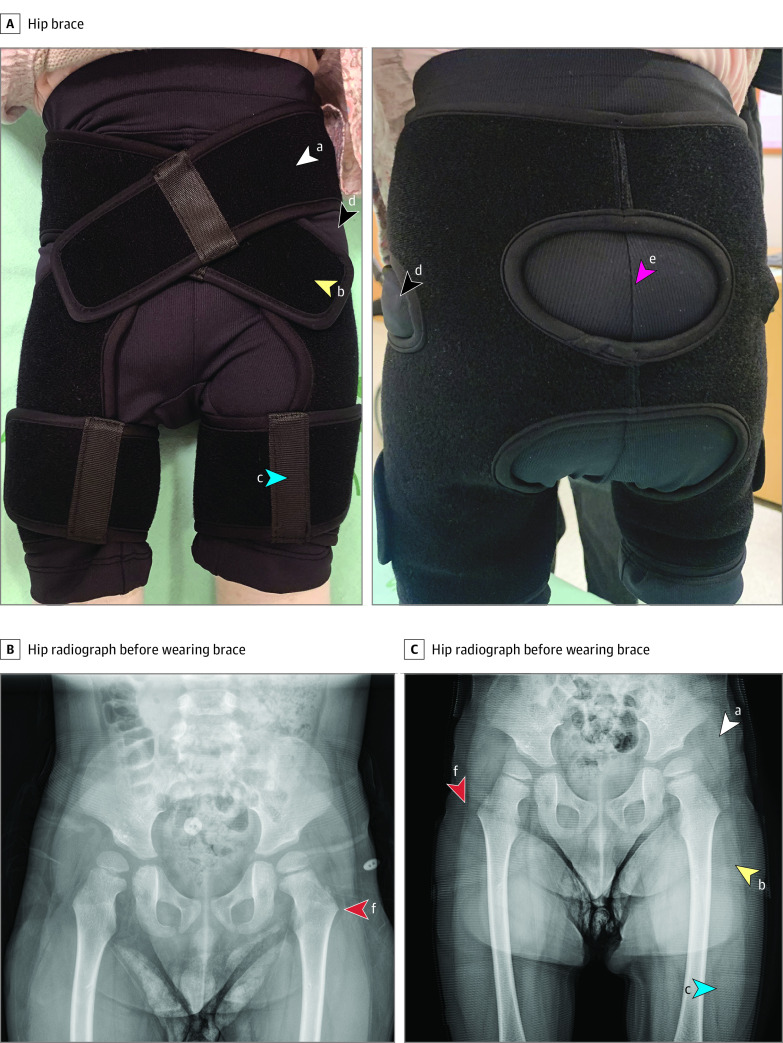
Hip Brace and Example Radiographs of a Patient Before and After Wearing the Hip Brace ^a^Upper straps were designed to protect hip joints from displacement. ^b^Lower straps were designed to prevent coxa valga. ^c^Thigh straps were designed to prevent hip adduction. ^d^To maximize the preventive effect on hip joint displacement, the greater trochanter (d) should be located between the upper and lower straps. ^e^The round design was applied at the buttock area of the fabric to allow comfort when lying or sitting and to prevent movement of the orthosis. ^f^The hip brace compresses the capsule and ligaments around the hip joints where displacement occurs, thereby helping with normal alignment.
